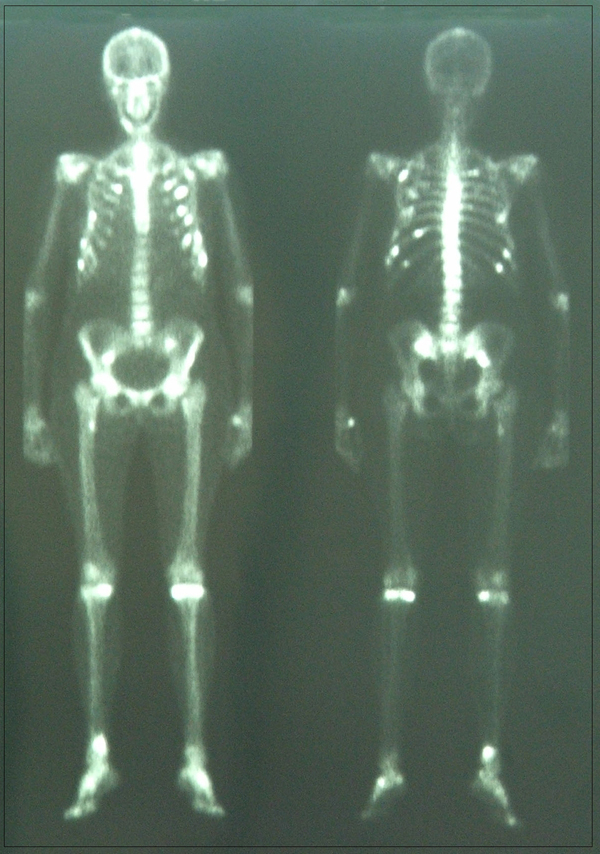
Whole body bone scintigraphy shows multiple foci of increased radiotracer uptake in the rib cage, the lumbar spine, the sacroiliac region, the bilateral knee and the right tibiotarsus.
